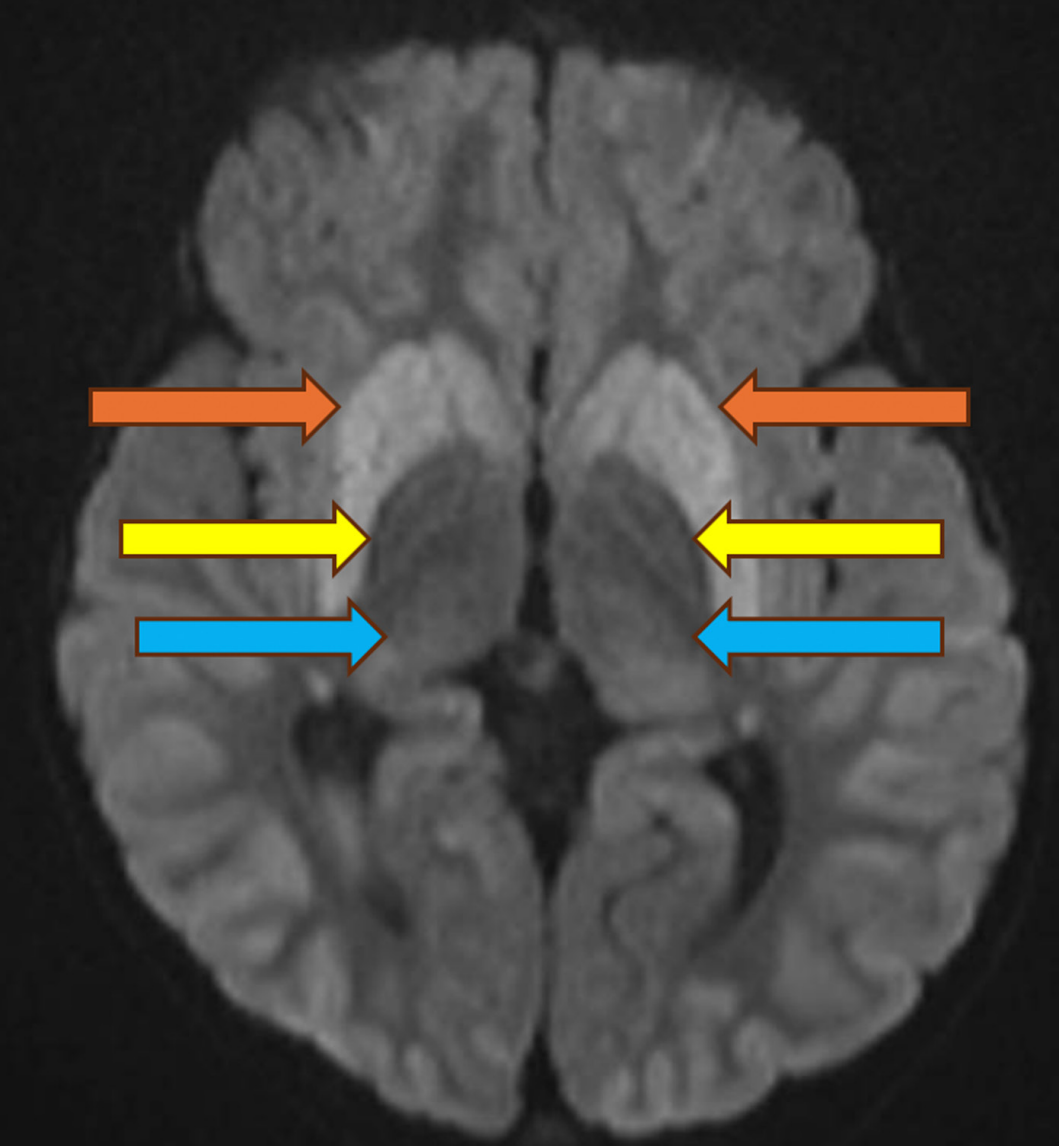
DWI demonstrating bilateral symmetric diffusion restriction in caudate nucleus and putamen (orange arrow), globus pallidus (yellow arrow) and thalami (blue arrow).
